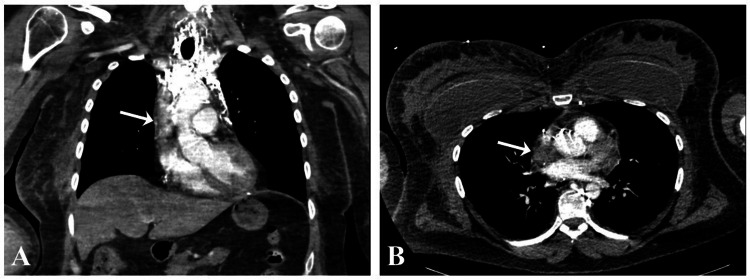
CT angiography of the chest showing SVC stenosis/high-grade partial obstruction with interval visualization of the numerous mediastinal venous collaterals in (A) coronal view and (B) axial view. CT: computed tomography; SVC: superior vena cava
